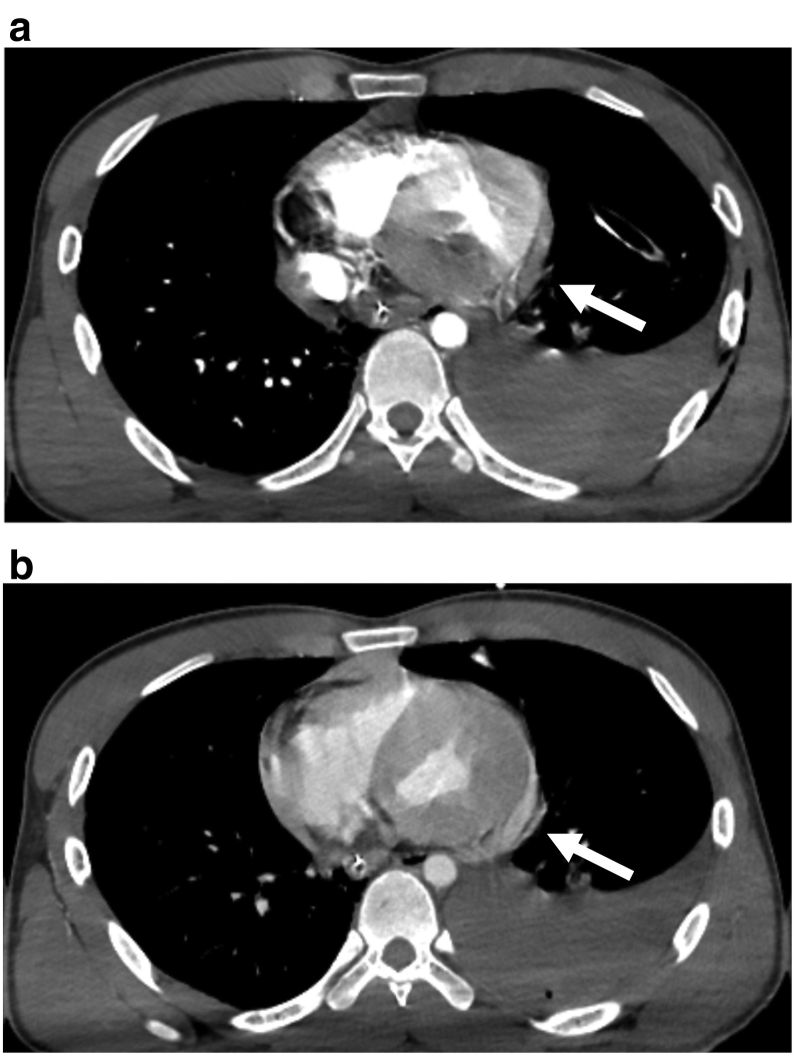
(a) Early-phase enhanced chest computed tomography showing a small pericardial effusion and left hemothorax. (b) Late-phase enhanced chest computed tomography showing an enhanced pericardial effusion compared with the early phase (arrows).
